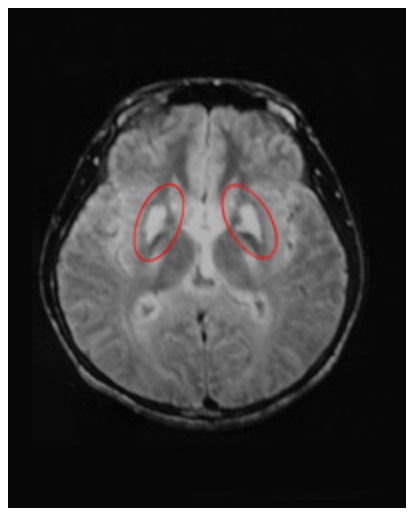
An MRI image of a typical WD subject. The image shows bilateral basal ganglion damage.
